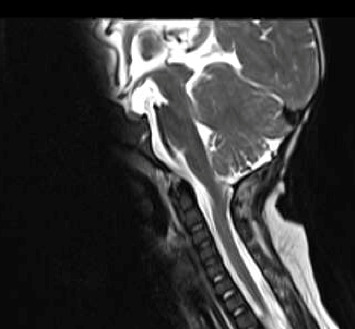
One-year postoperative T2 weighted sagittal MRI demonstrating complete resolution of cervical syringomyelia (from patient notes).
